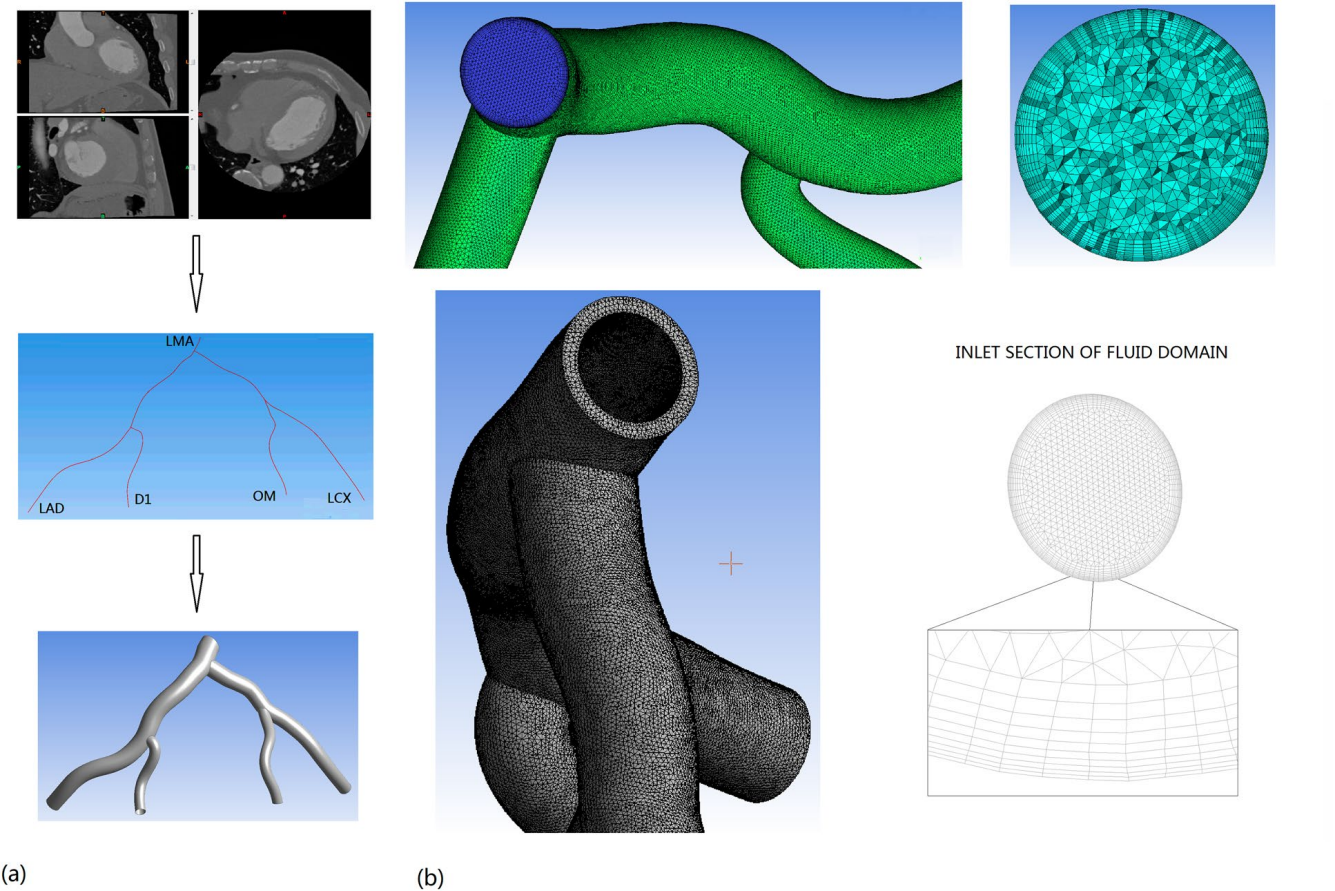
Reconstruction of 3D human left coronary artery. (a) Reconstructed human left coronary artery from the original *CT* coronary data of a 54-year-old male patient. *LMA* left main coronary artery, *LAD* left anterior descending artery, *LCX* left circumflex artery, *D1* first diagonal branch of the *LAD*, *OM* left obtuse marginal branch. (b) Meshes of blood-phase & arterial wall of human left coronary artery.
